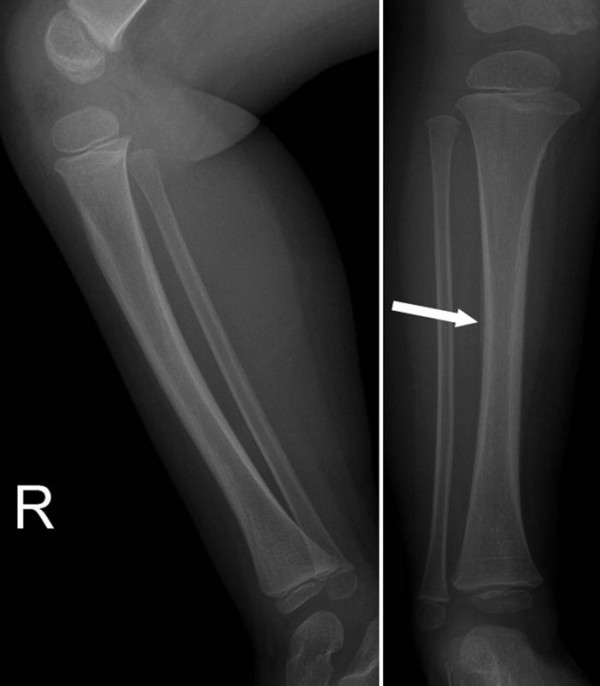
Lower extremity radiograph showed periosteal new bone formation suggesting healing of a toddler's fracture.
